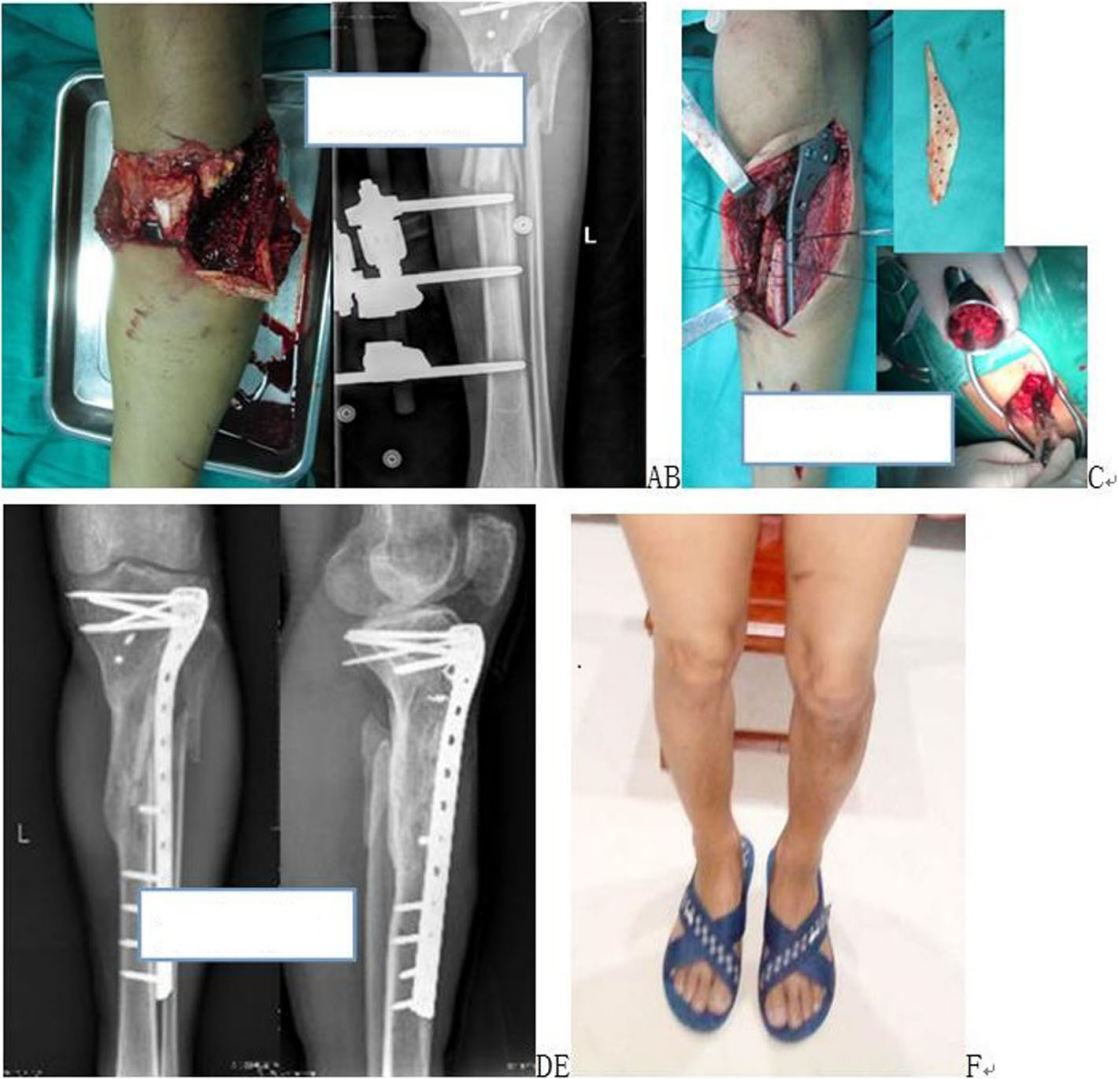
A patient with open upper tibial fractures combined with bone defects treated with line binding cortical bone wrapped cancellous bone graft. a and b Wound appearance and X-ray films of external fixation and reserved free cortical bone. c Surgical procedure using line binding cortical bone and the wrapped cancellous bone graft technique in second intention surgery. d and e X-ray films show bone healing preoperative 10 months. f Appearance 18 months postsurgery
